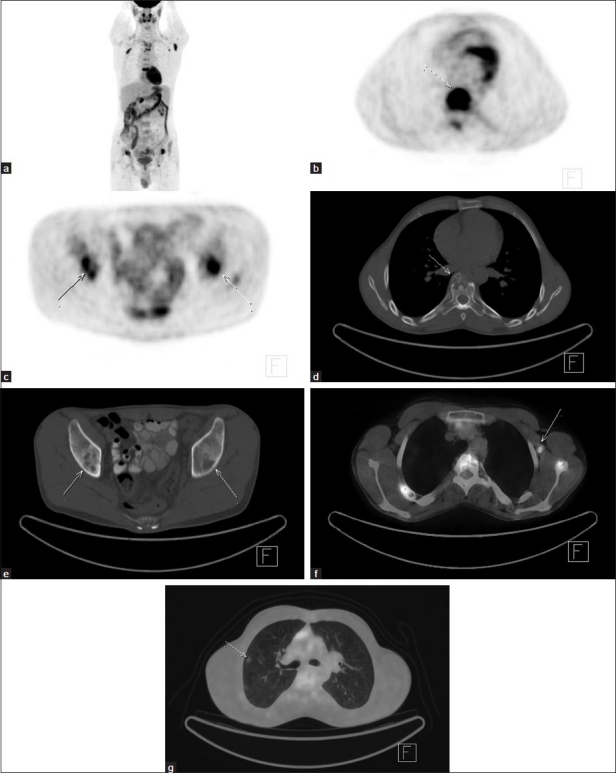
PET/CT imaging revealed intense FDG uptake in dorsal 2, dorsal 8, right second rib, left scapula, sternum and bilateral iliac bones (a, MIP, b and c, axial PET images) which were litic in CT slices (d and e, axial CT images). Maximum standard uptake value of bone lesions was 9,6. In addition to bone lesions FDG uptake in left axillary lymph node with a maxium standard uptake of 5,4 (f, axial fusion image) and minimal FDG uptake in right upper lobe lung pulmonary nodule with a maxium standard uptake of 2,1 were seen (g, axial fusion image)
